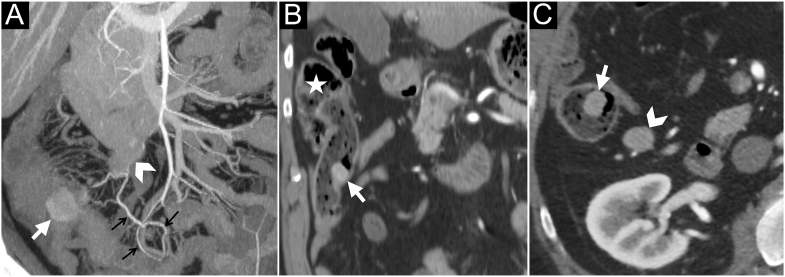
CT signs evaluated for the need of a right colectomy. (A) The patient is a 55-year-old woman. Arterial phase – coronal oblique MIP reconstruction. The mesenteric mass (arrowhead) involves the ileocolic artery, which is very irregular, and there is an arterial supply network (small black arrows) from the ileal branches. The Si-NET (white arrow) is located near the ileocecal valve. (B) The patient is a 66-year-old man. Arterial phase – coronal reconstruction. The Si-NET (white arrow) is located near (<10 cm) the ileocecal junction and the cecum (white star). (C) The patient is a 66-year-old man. Arterial phase – axial. Presence of a LN metastasis in the right mesocolon (white arrowhead), adjacent to a distal Si-NET (white arrow).
